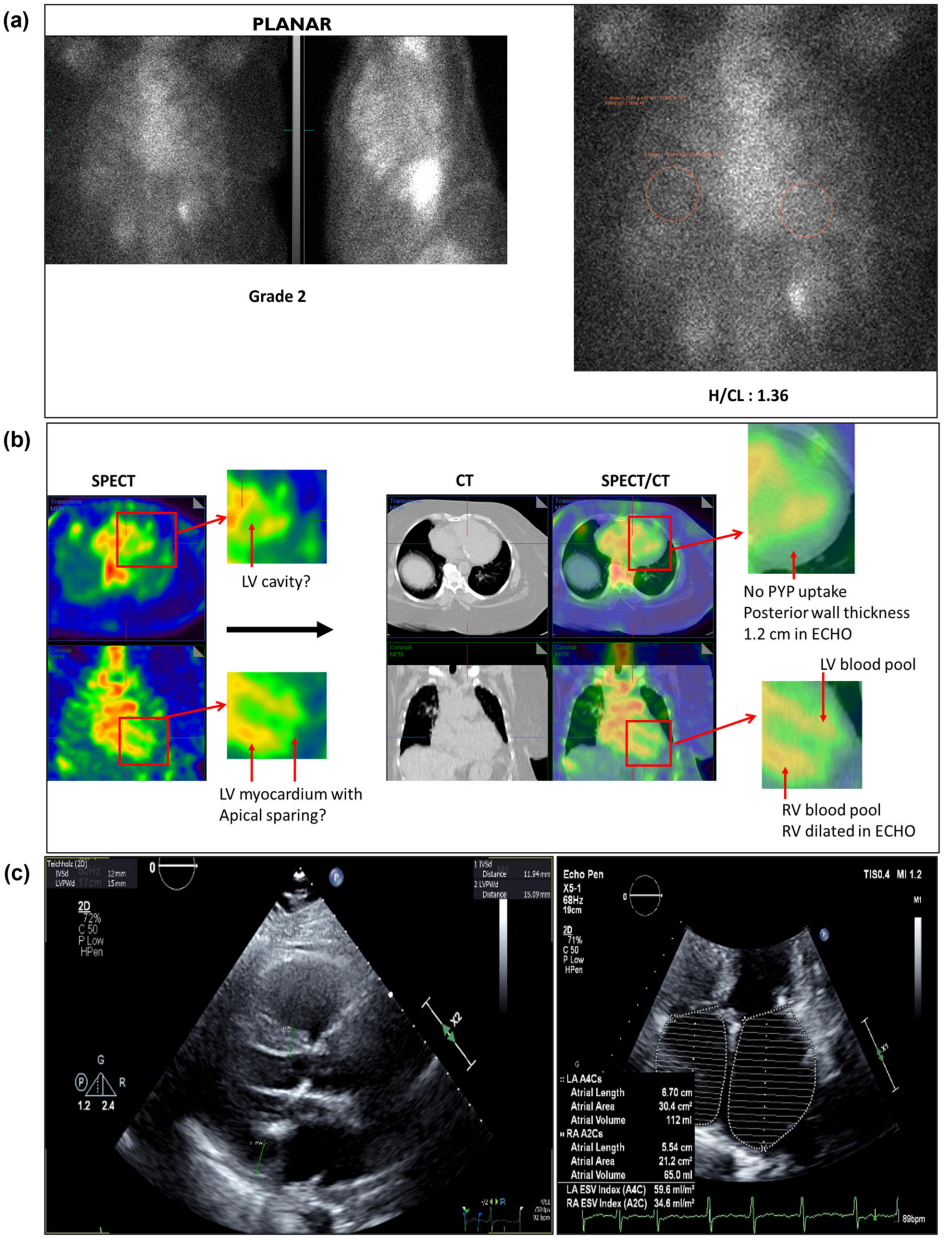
Pseudo-apical sparing (A) Three-hour planar imaging shows Grade 2 tracer uptake and H/CL ratio of 1.36; interpreted as “strongly suggestive of ATTR amyloidosis”.^5^ (B) SPECT appears to demonstrate LV wall tracer uptake, sparing the apex. SPECT/CT shows no PYP uptake in the myocardium, but rather confirms LV and RV blood pool. Note dilated RV and thickened posterior wall (1.2 cm). SPECT/CT reclassifies case from strongly suggestive to not suggestive. (C) Four chamber ECHO clearly indicates enlarged RA and RV. Posterior wall is 1.2 cm, septum is 1.2 cm
